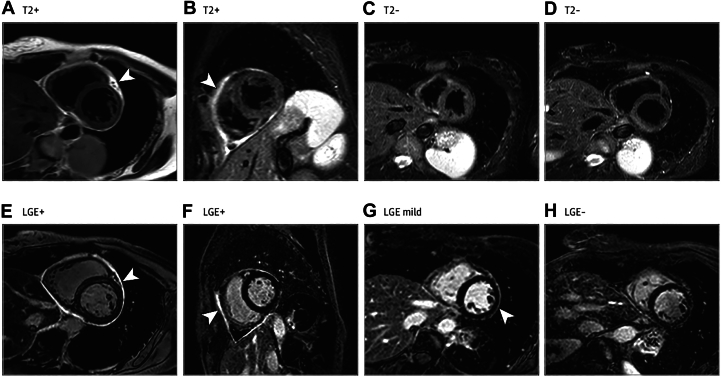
Cardiac Magnetic Resonance Findings in Recurrent Pericarditis Cardiac magnetic resonance showing an increased signal intensity on T2-weighted sequences representing pericardial edema (A and B) and pericardial late gadolinium enhancement (LGE) and inflammation (E and F) with interval improvement on anti-inflammatory therapy (C and G) and resolution (D and H). Adapted with permission from Kumar et al.^16^
